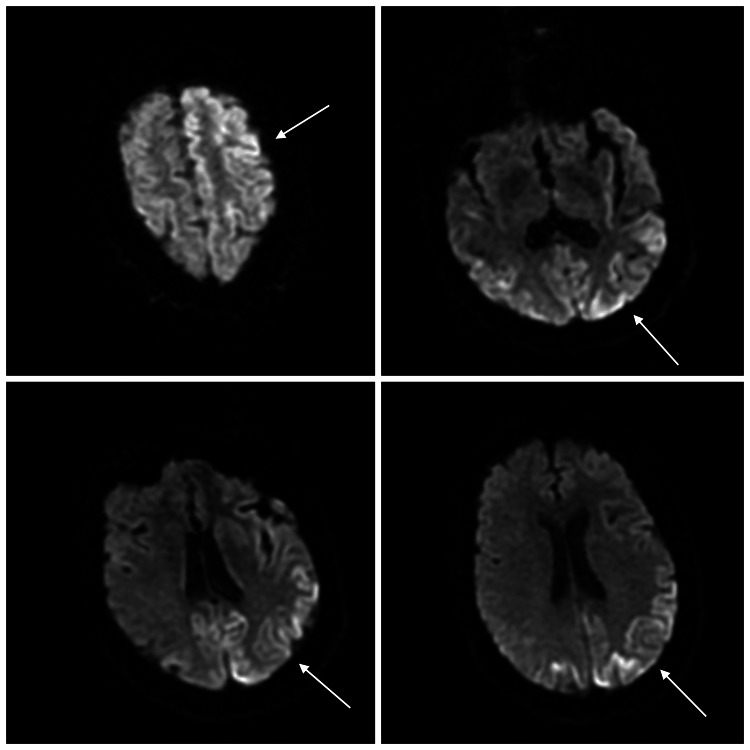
Diffusion-weighted sequence (DWI) MRI transverse section of brain showing gyral areas of restricted diffusion in left frontal, parietal, temporal, and occipital regions
